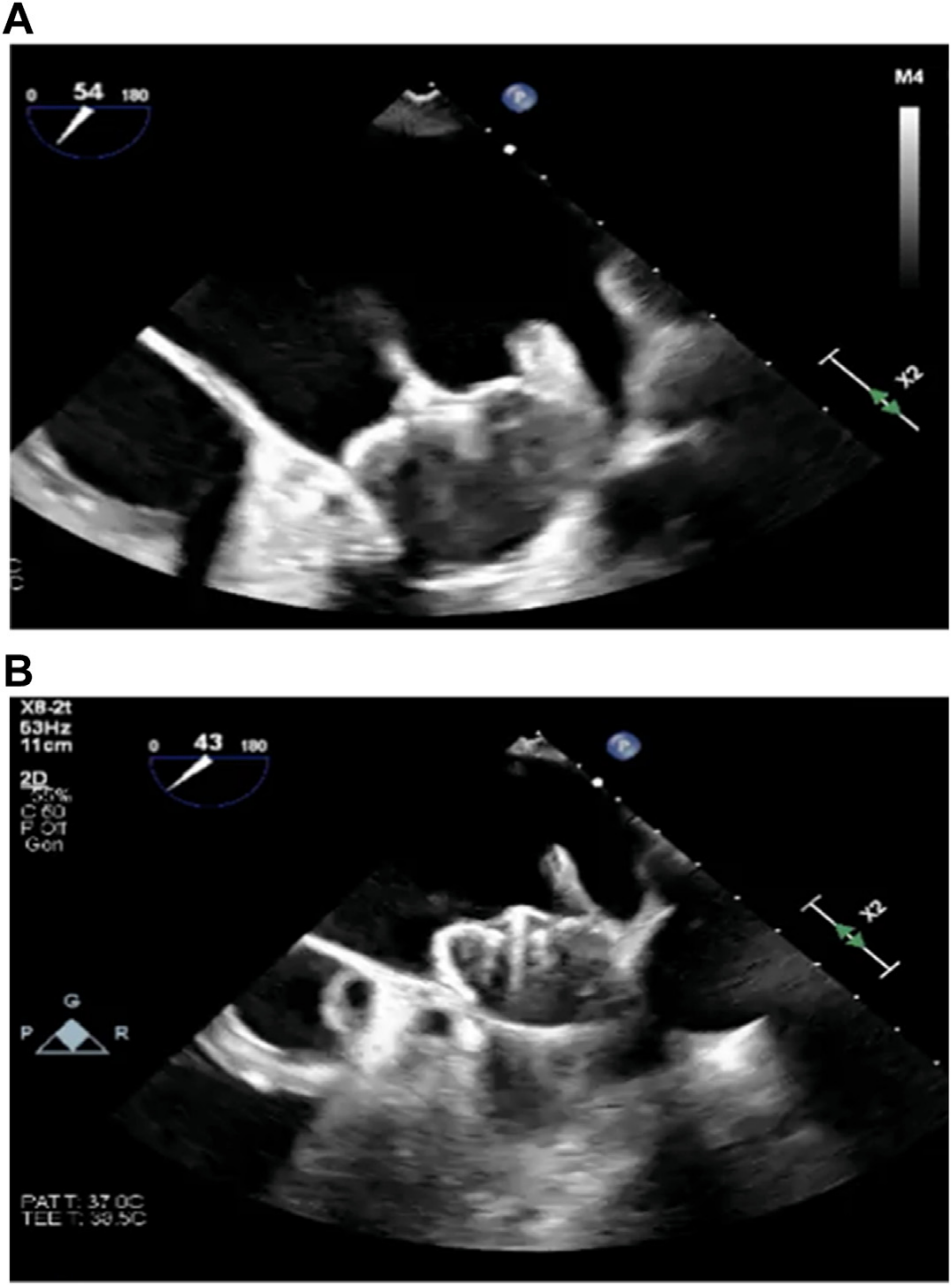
Device Tilting Seen With Significant Coumadin Ridge Edema at the Landing Zone (A) TEE at the time of device implant. (B) TEE at 95 days post-implant. TEE = transesophageal echocardiogram.
